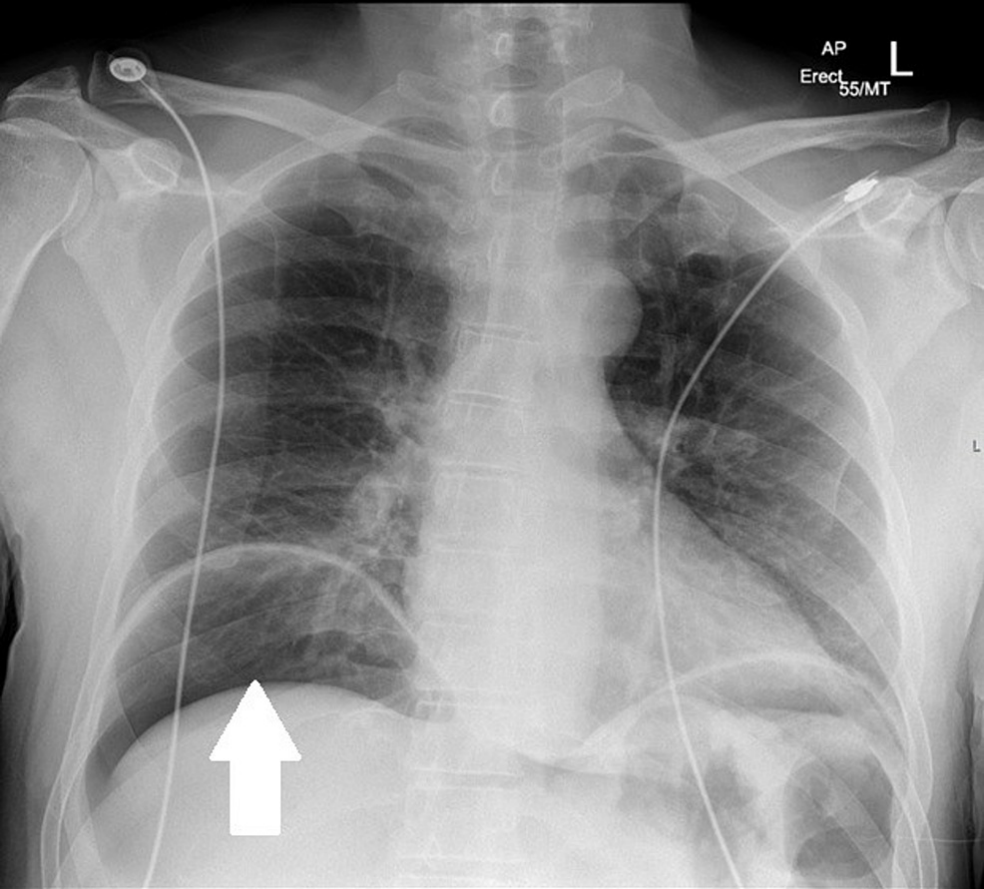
Erect CXR is better than AXR in the detection of the pneumoperitoneum (see white arrow). CXR: chest X-ray; AXR: abdominal X-ray.
